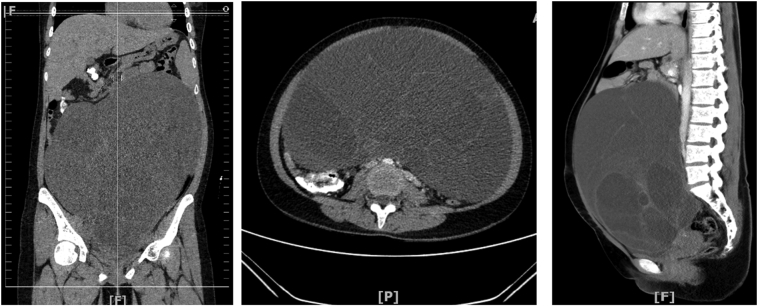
CT whole abdomen with contrast showed a large cystic multilocular septated lesion with mucinous fluid.
